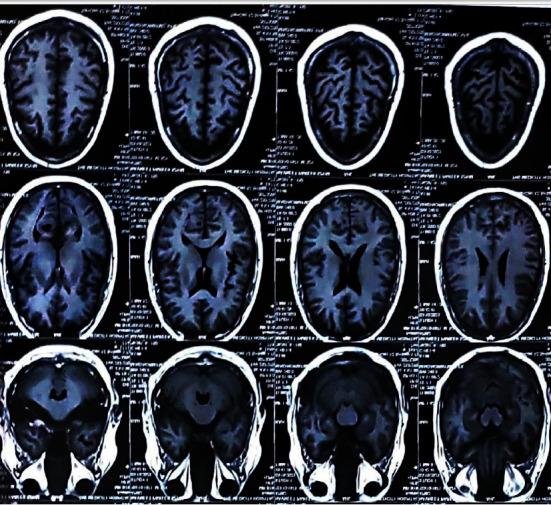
Normal study: MRI brain.
